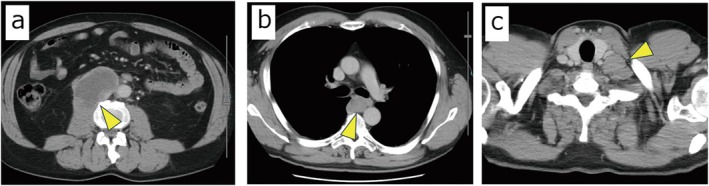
Contrast‐enhanced tomography (CT) revealed metastases to the left supraclavicular (c), mediastinal (b), and para‐aortic lymph nodes (a), accompanied by right ureteral obstruction. No other organ metastases were detected.
